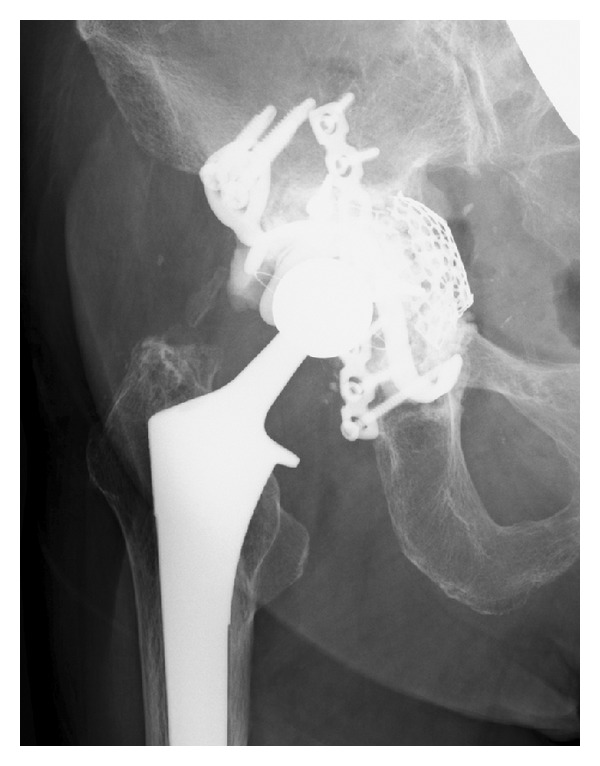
Latest radiograph of the hip taken 3 years after the operation. There is no migration or loosening of the prosthesis.
